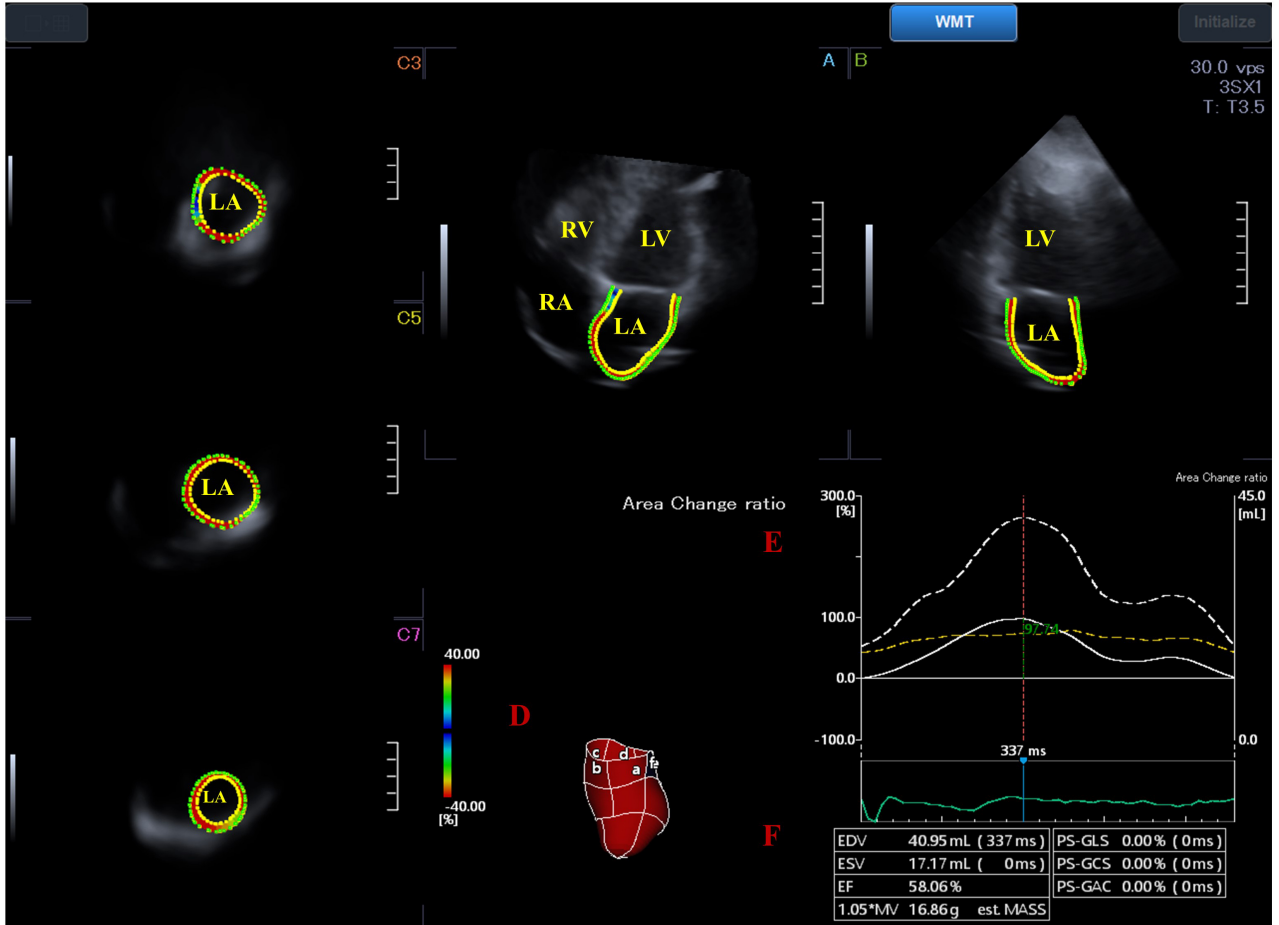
Three-dimensional (3D) speckle tracking echocardiography-derived
